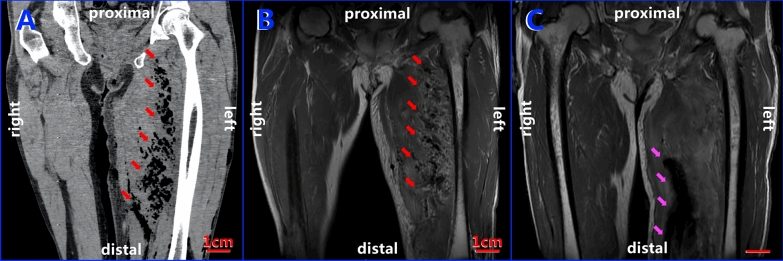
CT and MRI images showing gas-producing infection area changes in the left upper thigh. A CT coronal plane of the left thigh showed massive amounts of gas visible in the muscle space (red arrows); B corresponding to the CT image, MRI showed massive gas signals in the muscle space (red arrows); C MRI showed gas in the left thigh that disappeared after adjustment of the antibiotic regimen according to the 16S rDNA sequencing results, with the pink arrows pointing to the VSD equipment inserted in the lesion
